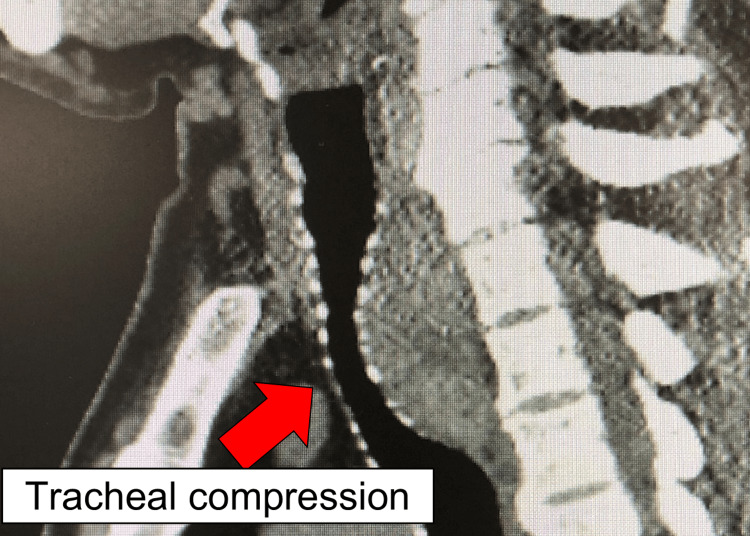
Non-contrast CT of the cervical region (sagital view) showing tracheal compression by an anterior mediastinal tumor.
